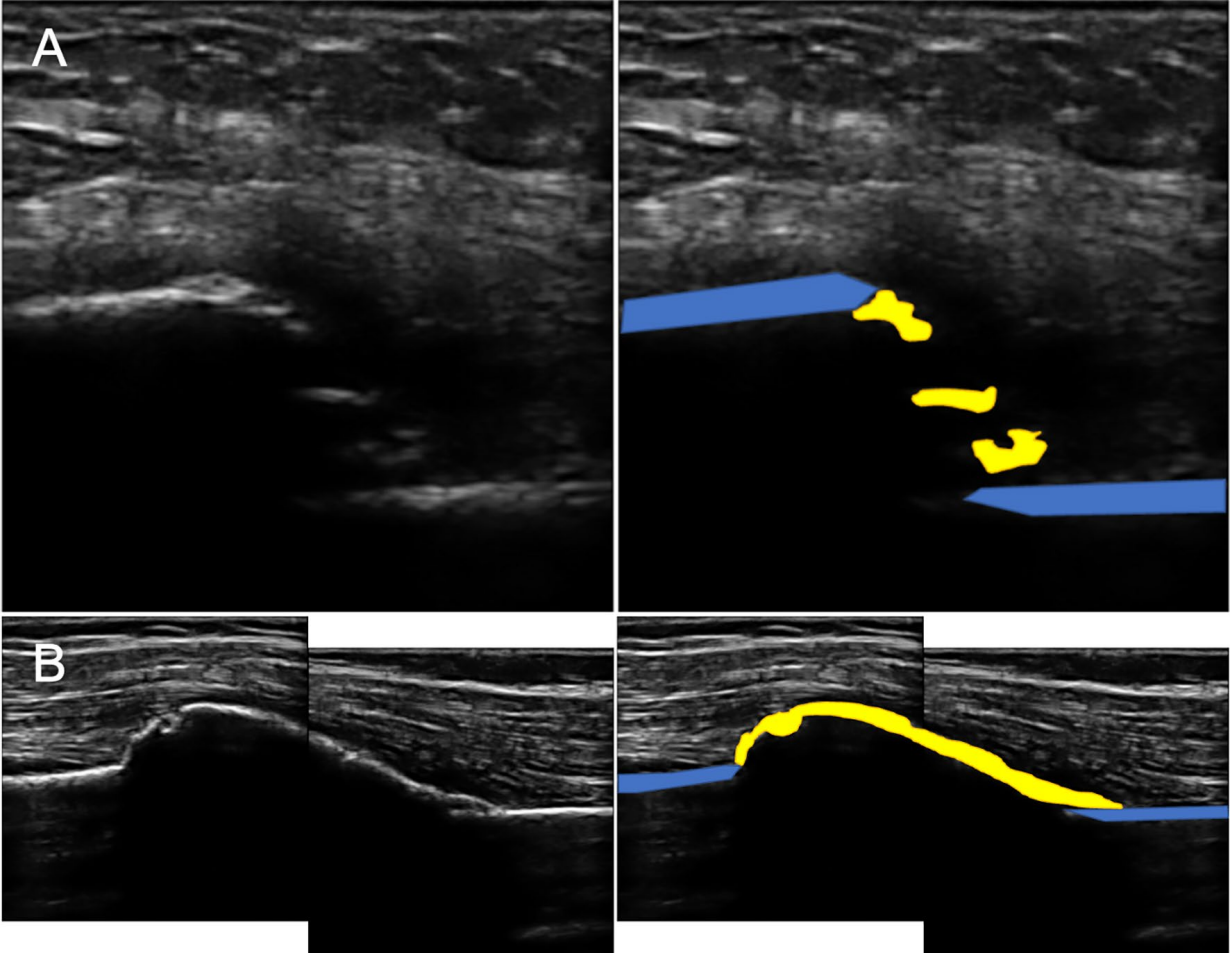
Ultrasound scan images obtained at six weeks following a humeral shaft fracture, showing: A Non-bridging callus; B Bridging callus; blue = bone cortex, yellow = sonographic callus
